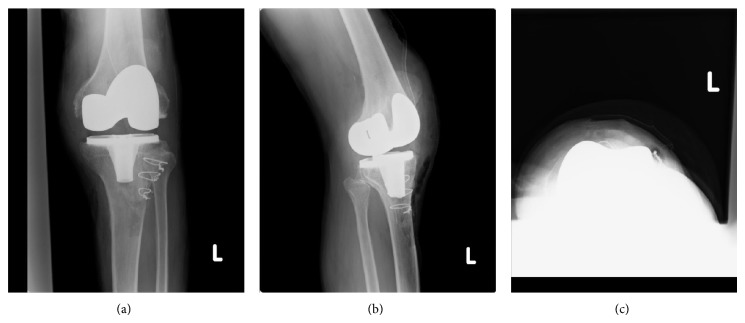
Postoperative radiographs: anteroposterior view (a), lateral view (b), and skyline view (c) of left knee.
